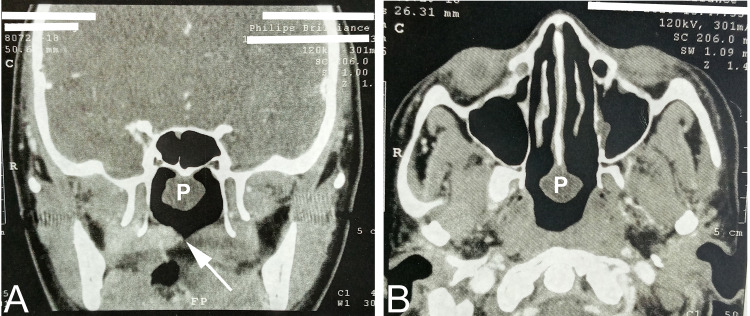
Preoperative computed tomographic views for the nasopharynx (A) A coronal view shows a polyp (P) in the upper part and the arrow points to a notched soft palate in the lower part of the nasopharyngeal cavity. (B) An axial view shows the polyp (p) originating from the posterior border of the nasal septum.
